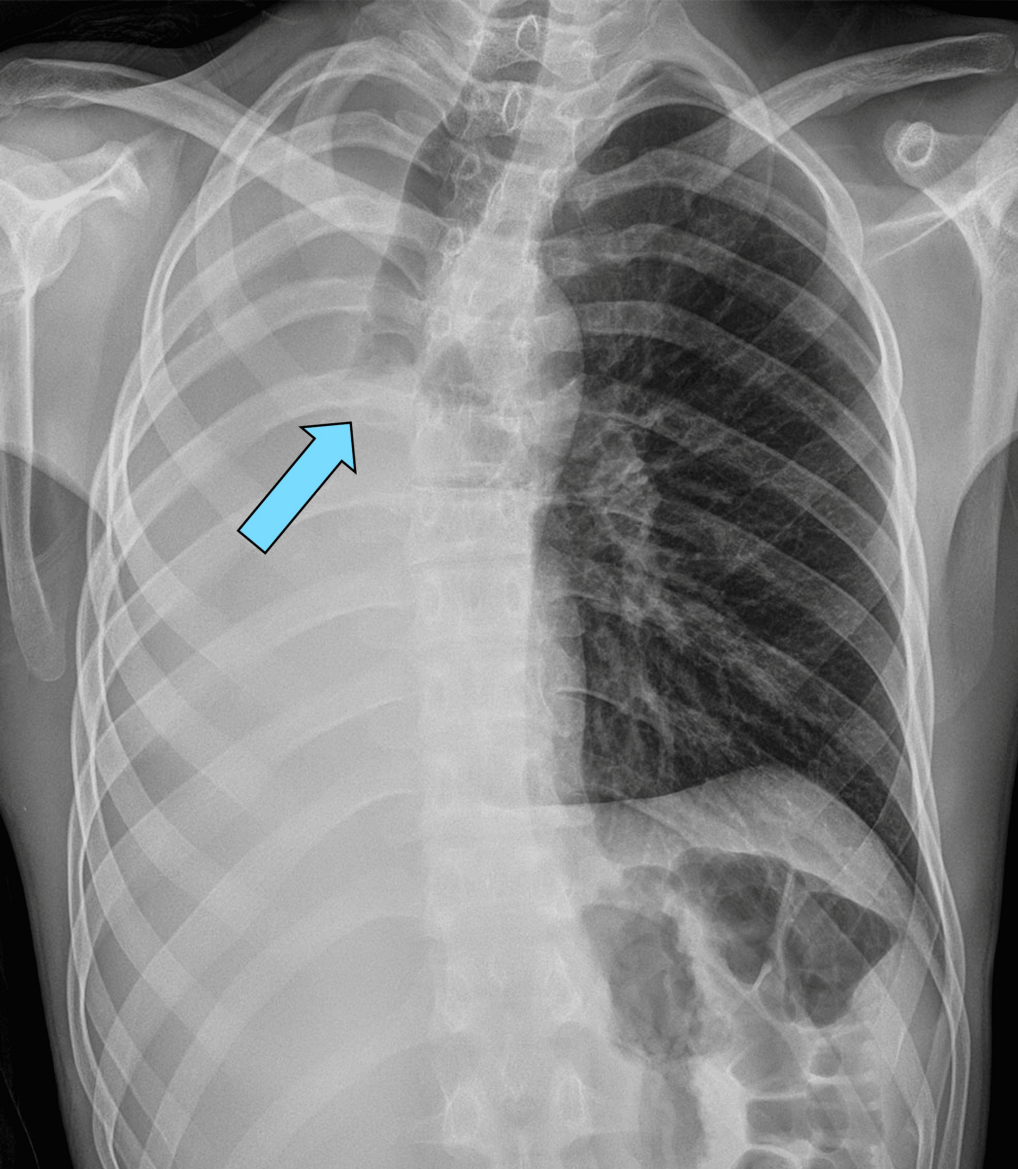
Postoperative chest X-ray demonstrating normal filling of the right pleural cavity following pneumonectomy. The image also shows the intact and well-sealed right bronchial stump (arrow).
